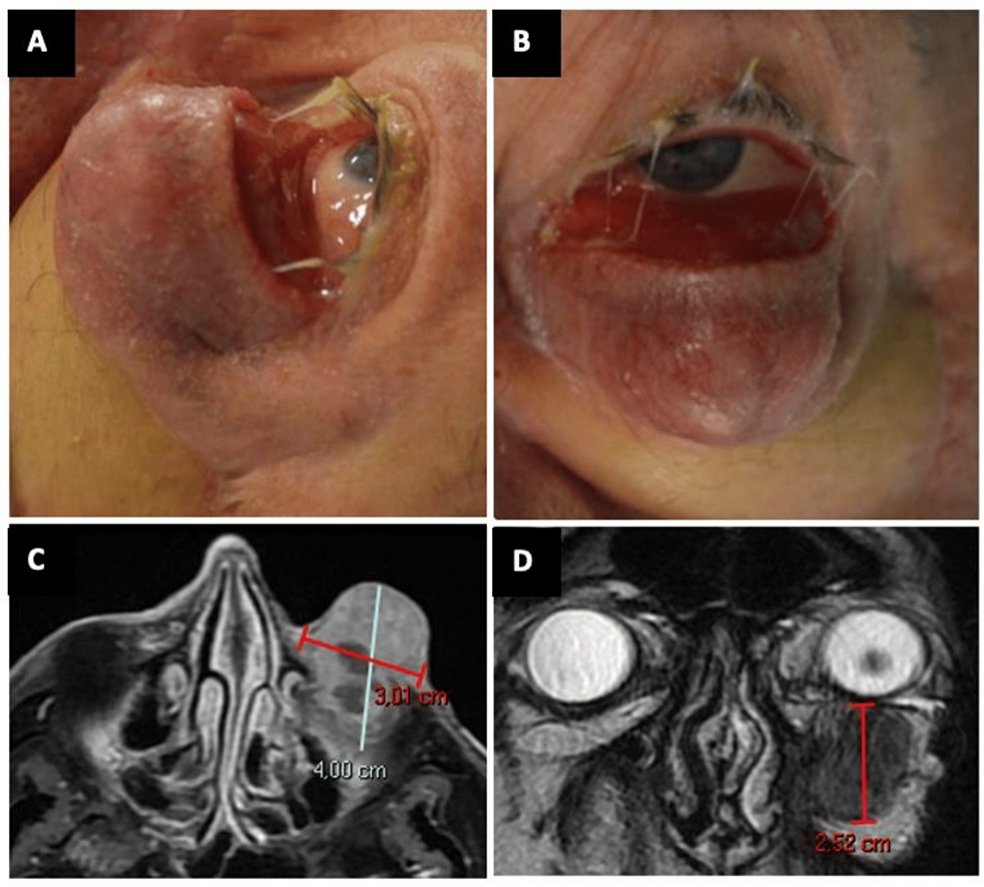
Clinical and radiological appearance of the local recurrence A, B. An amelanotic large mass involving the left conjunctiva and orbit; C, D. MRI of the left orbit showing orbital and maxillary involvement Axial (left) and coronal (right) T2-weighted spin-echo sequence shows a mass measuring 4.00*3.01*2.52 cm. MRI: Magnetic Resonance Imaging. Measures: anteroposterior*transverse*craniocaudal.
